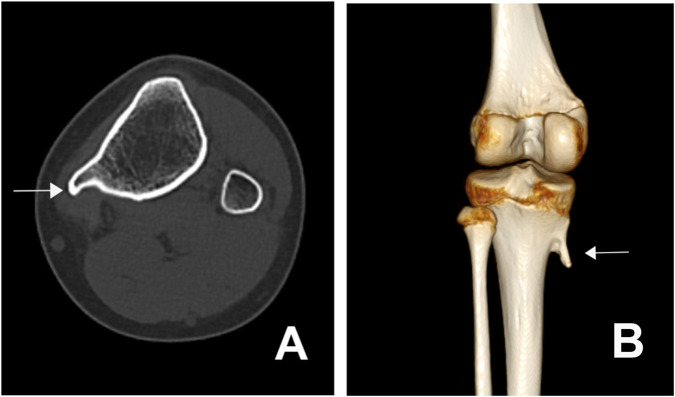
CT imaging of osteophyte formation at the knee joint. (A) Axial CT scan of the knee joint shows an osteophyte (white arrow) at the proximal tibia. (B) Sagittal 3D reconstructed CT image demonstrates the osteophyte (white arrow) projecting from the proximal tibia at the knee joint.
